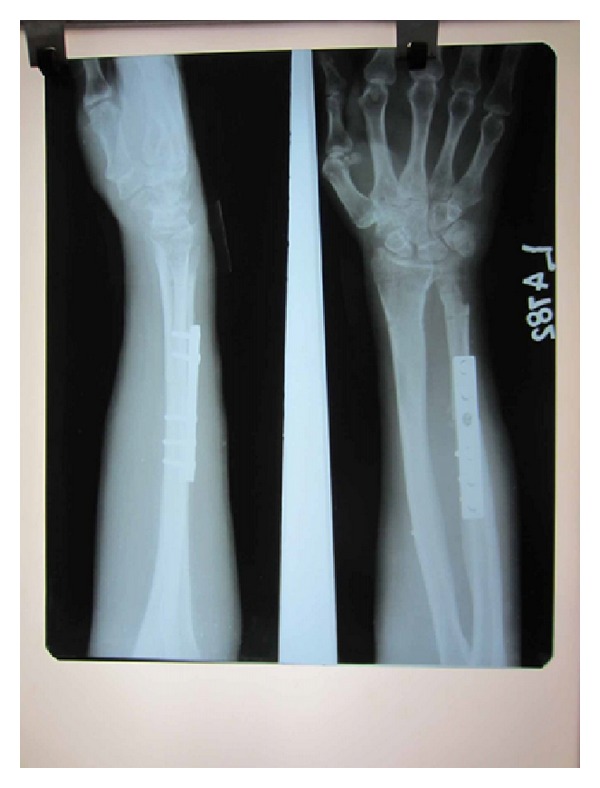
X-ray at 1-year followup.
